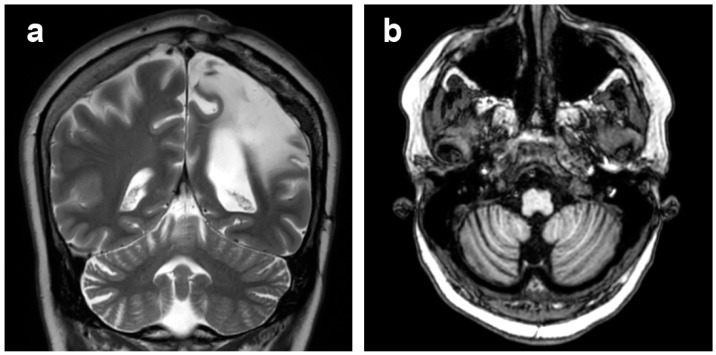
Patient 1, cerebellar diaschisis. (a) Coronal T2-weighted image and (b) axial T1-weighted image, showing right cerebellar atrophy contralateral to the affected cerebral hemisphere.
